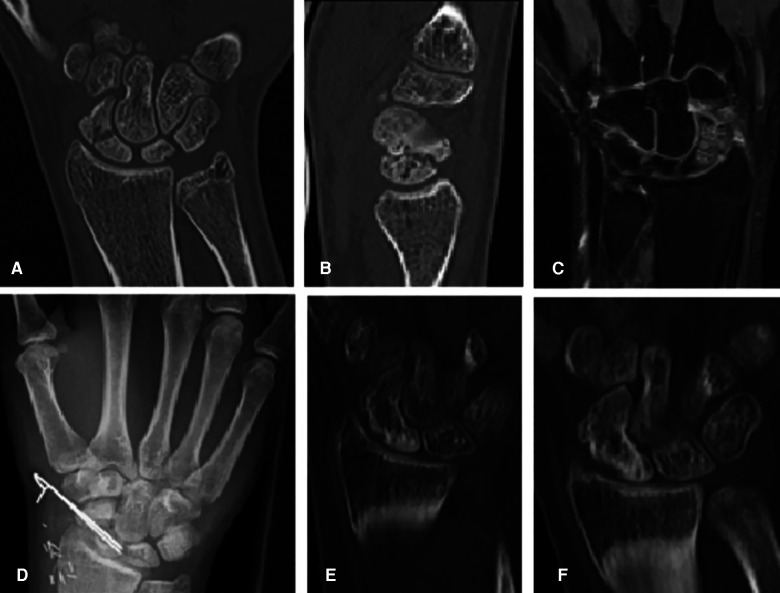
(A) Coronal CT scan showing the mid-waist of an 18-year-old male patient with a scaphoid nonunion 1 year after sustaining a scaphoid fracture, which initially remained undetected. (B) Sagittal CT scan. (C) Coronal gadolinium-enhanced MR imaging scan showing avascular necrosis of the proximal pole. (D) Dorsopalmar radiograph postoperative after implantation and Kirschner wire fixation of a vascularized bone graft from the medial femoral condyle. (E) Coronal CT scan 3 months postoperative showing complete healing. (F) Coronal CT scan 6 months postoperative showing complete healing. MFC, medial femoral condyle; K-wire, Kirschner wire; MR imaging, magnetic resonance imaging.
